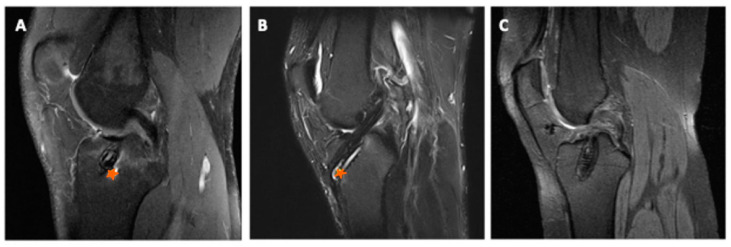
Different sagittal MRI scans showing bone marrow oedema ((A), orange star), tunnel liquid effusion ((B), orange star) and no signs of oedema or liquid effusion (C).
